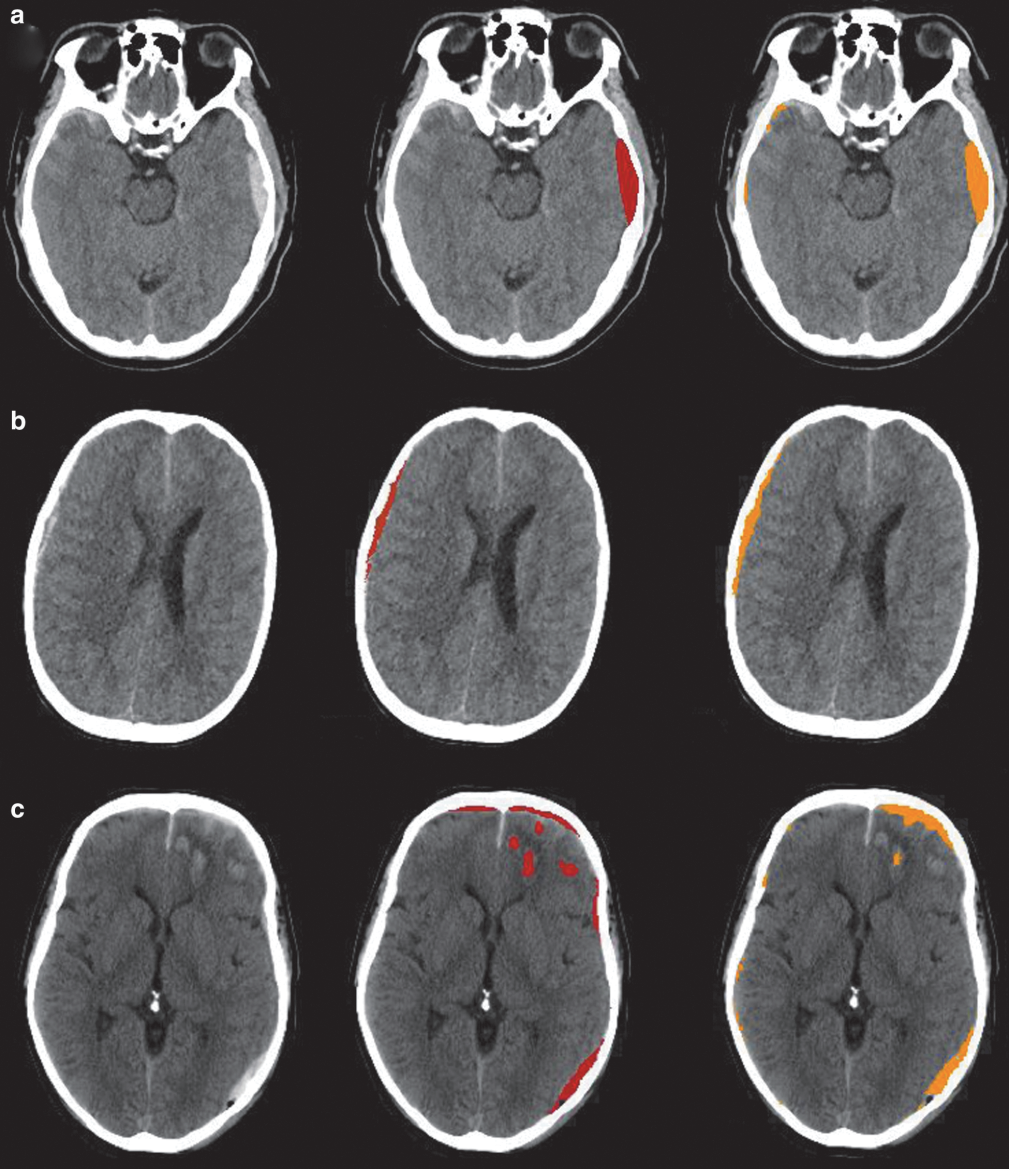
Qualitative results for acute intracranial lesions segmentation on 3 representative subjects from data set 1. The first column shows original computed tomography images with superimposed acute intracranial lesions segmentations from the expert in the second column and icobrain in the third column. (A) Acute intracranial lesions segmentation on a representative subject with Dice of 0.81, followed by (B) Dice of 0.73 and (C) Dice of 0.54. Color image is available online.
